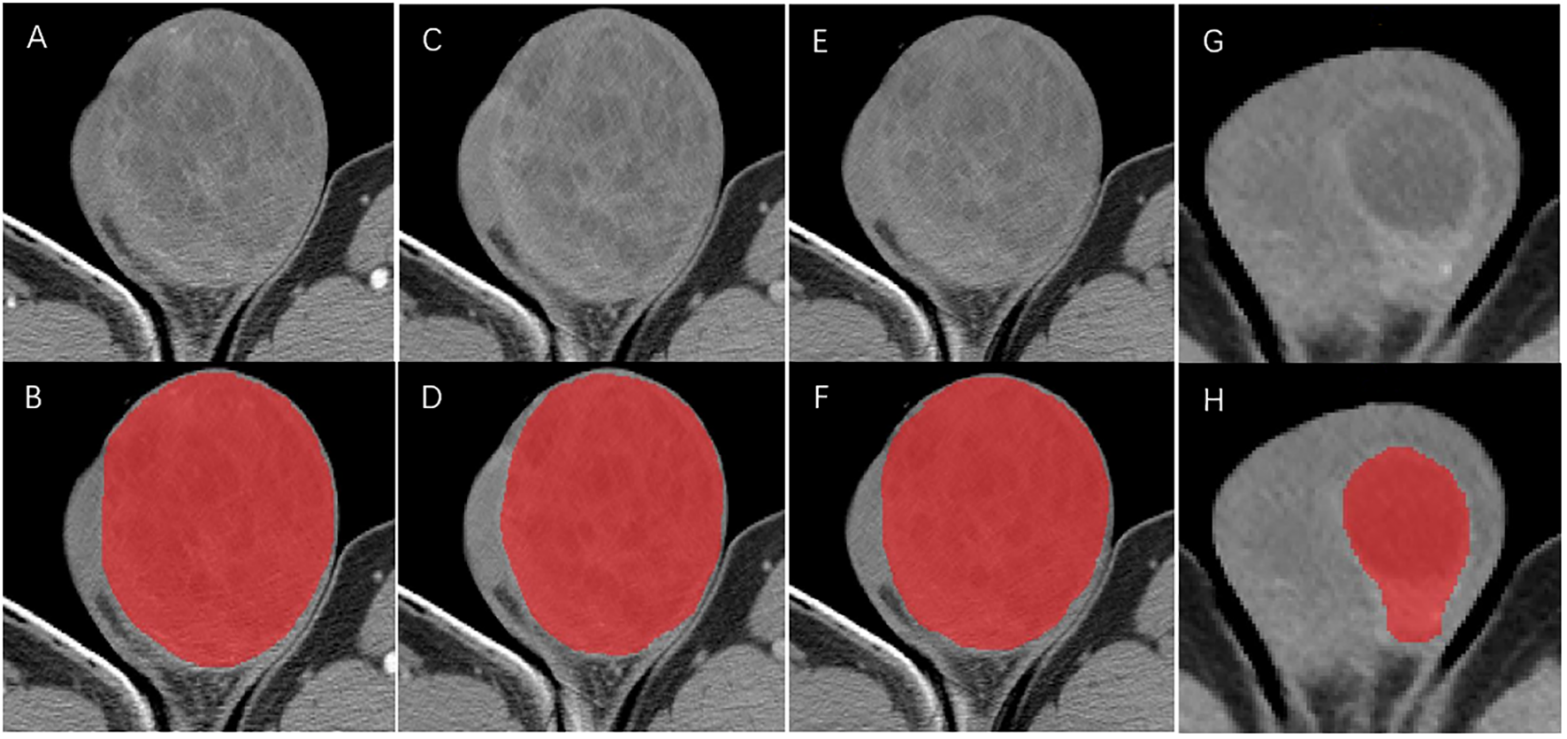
ROIs delineation in arterial phase (A, B), portal venous phase (C, D) and unenhanced phase (E, F) CT for the mass without a distinct border. ROIs delineation in arterial phase (G, H) for mass with a distinct border.
